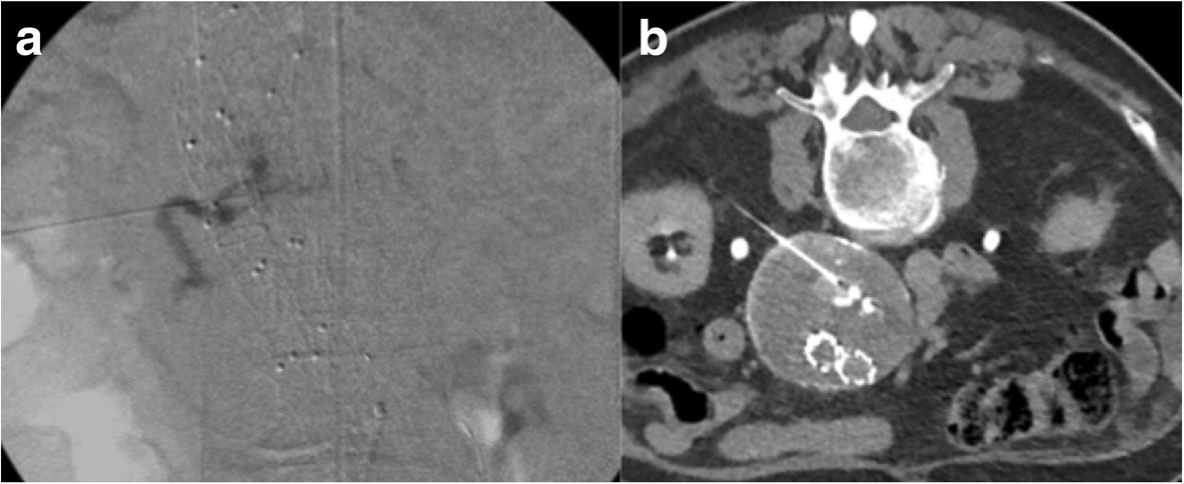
a Direct puncture of the sac under CT and fluoroscopic guidance. Angiogram confirms the presence of the small nidus and the feeding vessel. b CT scan during embolisation with Onyx
